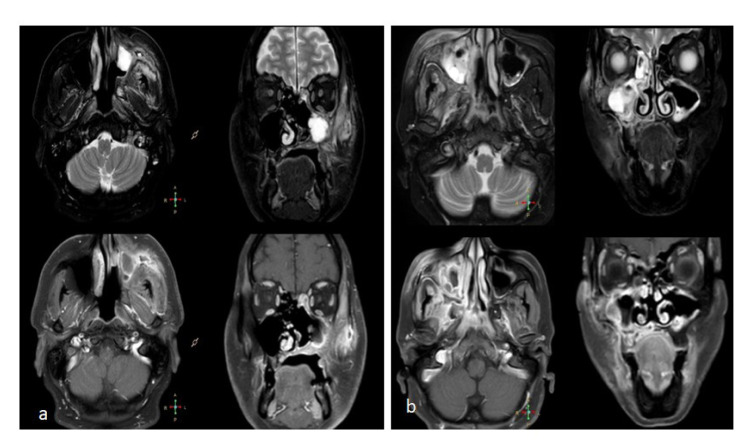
A) Axial and coronal T2 fat Sat and T1 contrast-enhanced magnetic resonance imaging (MRI) images showing enhancing polypoidal left maxillary sinusitis with pre-antral, retro-antral, and left facial soft tissue inflammatory changes. There is an extension along the left nasolacrimal duct. B) Axial and coronal T2 fat Sat and T1 contrast-enhanced MRI images showing enhancing polypoidal bilateral maxillary (right and left) and right ethmoid sinusitis with right pre-antral, retro-antral, and facial soft tissue inflammation. There is an intra-orbital extension along the floor of the right orbit.
